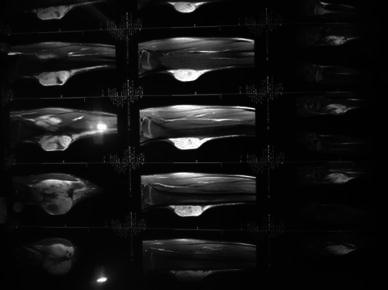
MR image showing heterogeneous, multilobulated, altered signal intensity mass lesion involving the muscular and fascial plane of the proximal left
